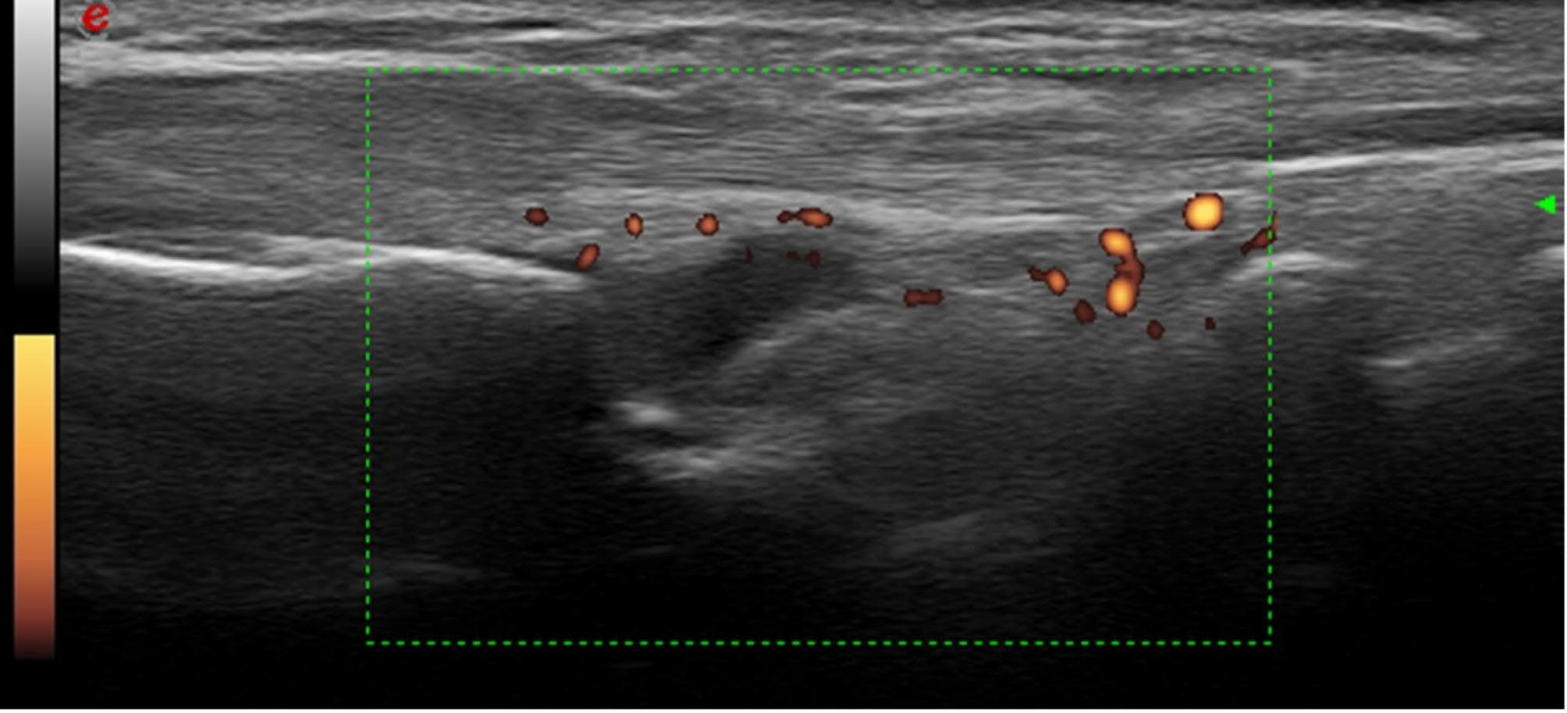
Ultrasound in a 40 -year-old woman with RA. The DAS28 is 2.6, while synovial hypertrophy is grade 2, power Doppler is grade 2
